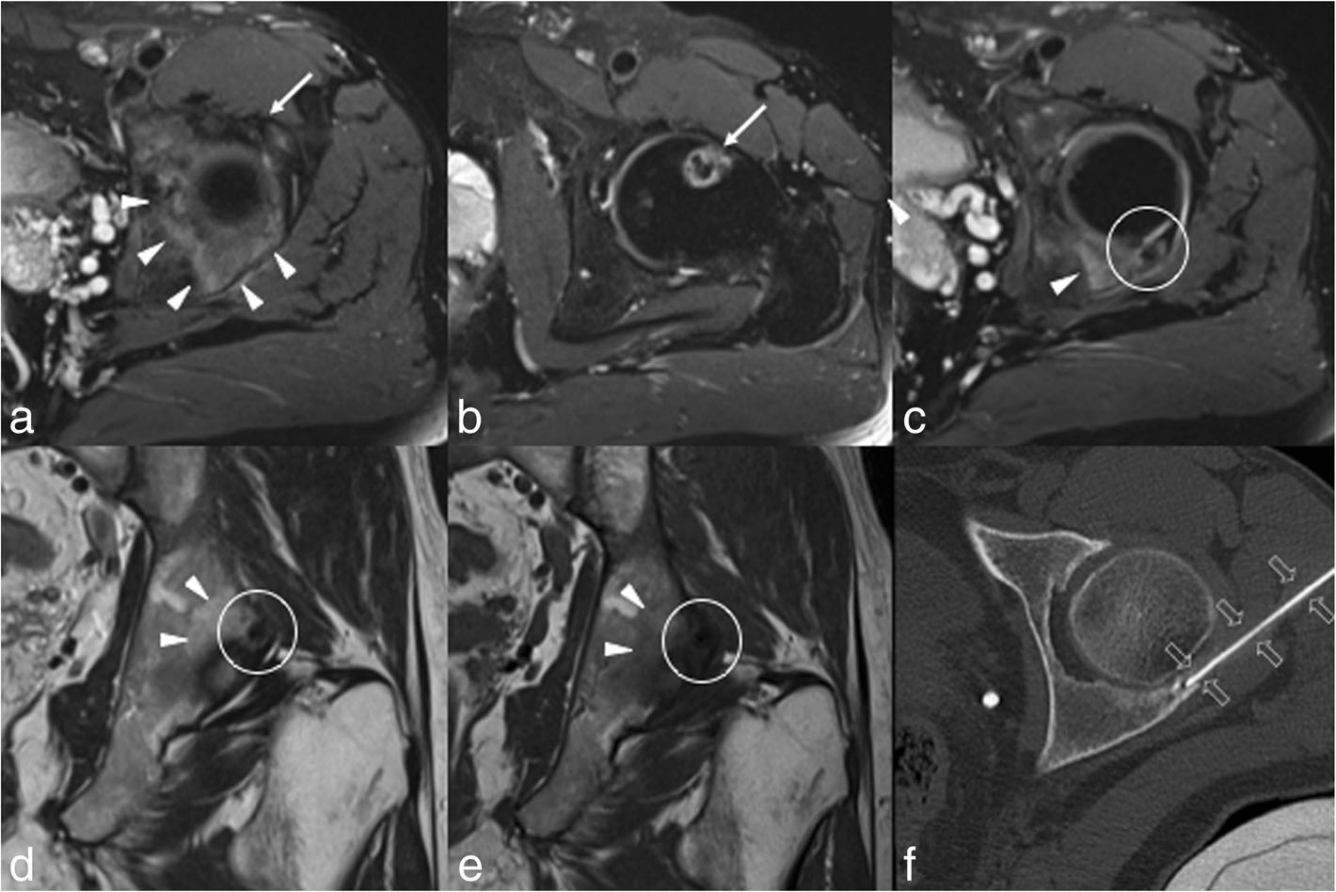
A few months after percutaneous RFA of both lesions and a partial pain relief, the patient complained increased left hip pain and underwent hip MRI (a-e). Axial fat-saturated proton density-weighted images show the good outcome of the RFA with no marrow oedema on the anterior acetabulum (a, arrow) and femoral neck (b, arrow). Axial fat-saturated proton density-weighted (c), coronal T2-weighted (d) and coronal T1-weighted (e) MRI images well depict the third bone lesion on the posterior acetabulum (circle) with extensive adjacent bone marrow oedema (arrowheads). Then, CT-guided percutaneous RFA (f, open arrows) of the lesion of the posterior acetabulum was performed
